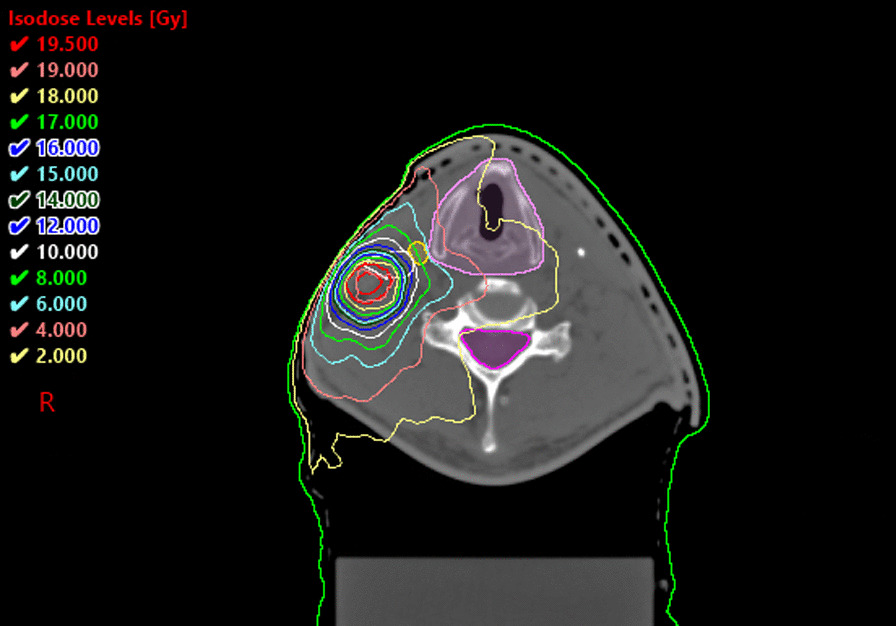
Stereotactic radiosurgery boost. Dose distribution shown on the CT scan. Isocenter plane of the stereotactic boost plan
